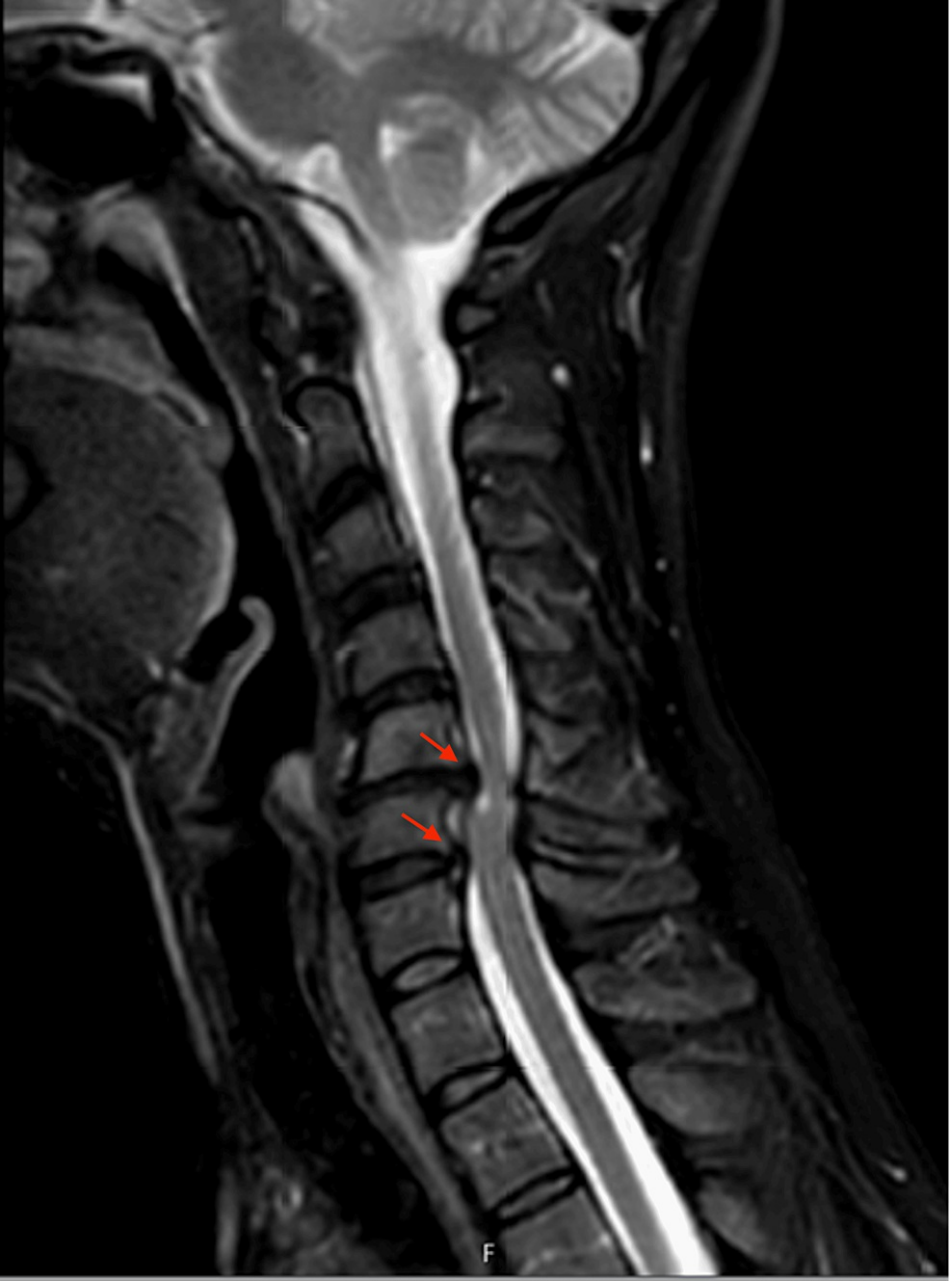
Sagittal T2 cervical MRI shows cervical myelopathy at C5-C6 and C6-C7 levels
